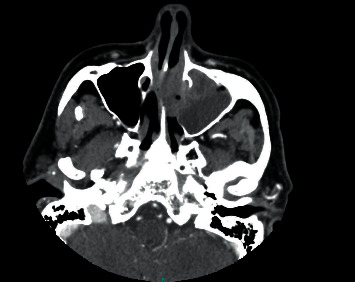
CT scan of the sinus showing an expansile mass in the left nasopharyngeal region leading to rightward nasal septum deviation and destruction of the etmoid cells.
